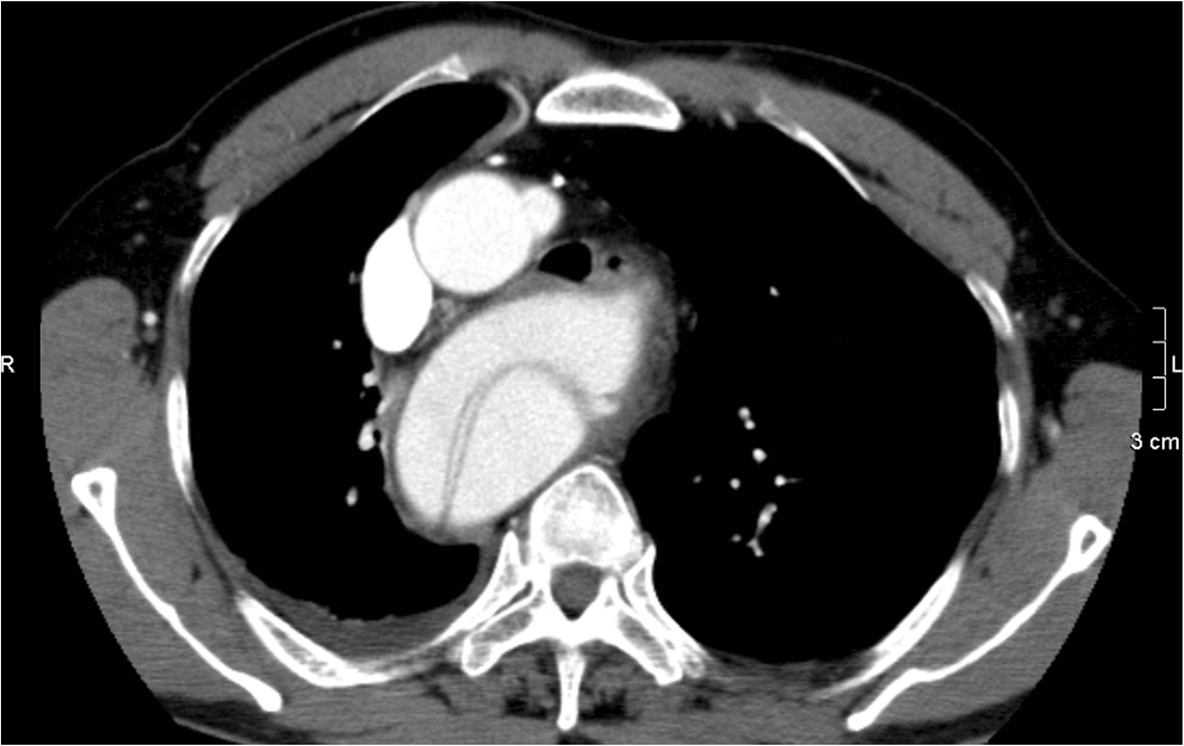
CTA demonstrated a right-sided aortic arch and a right descending thoracic aorta, a type B aortic.
